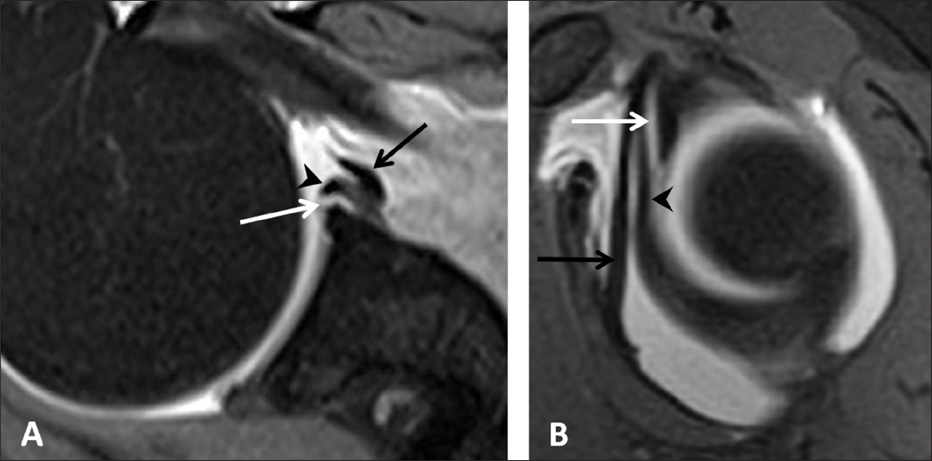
Sublabral foramen (sublabral hole). (A) Axial and (B) Sagittal fat suppressed T1-weighted MR arthrogram of a sublabral foramen. Sublabral foramen is located between the one o’clock and three o’clock position and provides a communication between the glenohumeral joint and the subscapularis recess (white arrows). Note the smoothly contoured, otherwise normal appearing anterior superior labrum (arrowheads), and middle glenohumeral ligament (black arrows) (Courtesy of Dr Deepu Alex Thomas).
